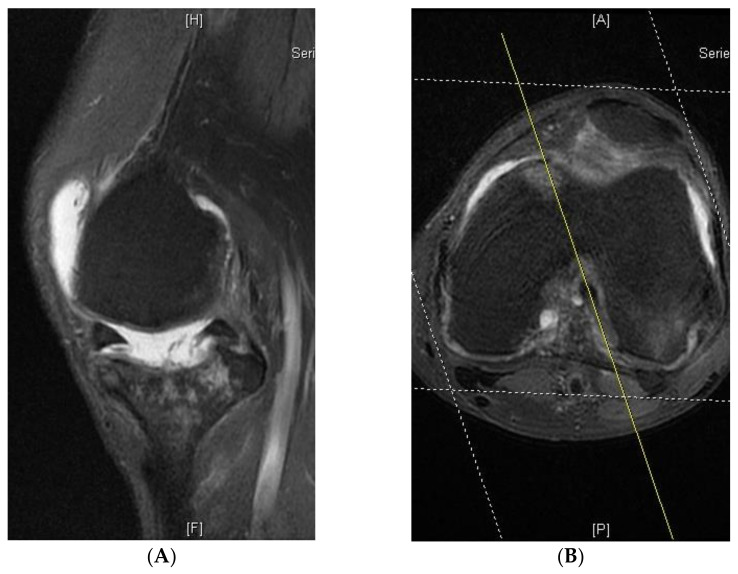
An unideal sagittal plane axis of magnetic resonance imaging would lead to incorrect measurement. (A) Sagittal cut of the MRI image. (B) Axial cut of the MRI image. The yellow line is the axis of the sagittal cut.
